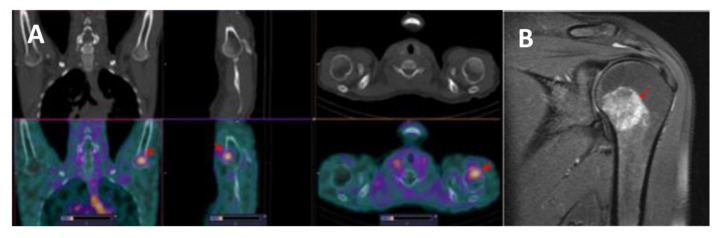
(A) SPECT/CT images of patient 11 at 2 h after injection of [^99m^Tc]Tc-TG. Elevated [^99m^Tc]Tc-TG uptake in the humerus head (SUVmax = 2.28) is visualized (arrows). No pathological changes were found using CT. The upper setting of the scale window (22% of the maximum number) was adjusted to visualize the lesion. (B) MRI image of the left shoulder joint, T1.
